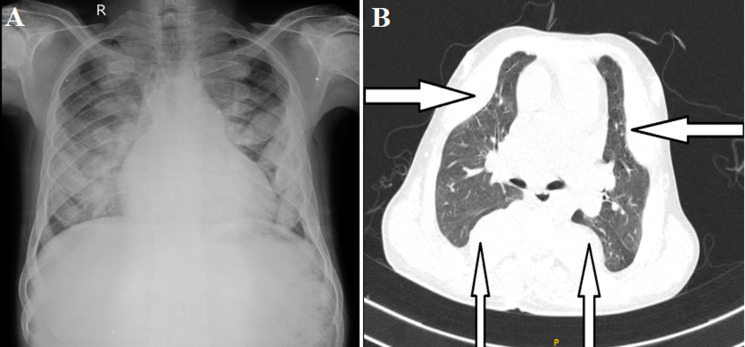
A) chest X-ray of the patient showing homogenous opacities with splaying of the ribs bilaterally; B) computed tomography of the thorax with white arrow showing sites of extramedullary hematopoiesis
